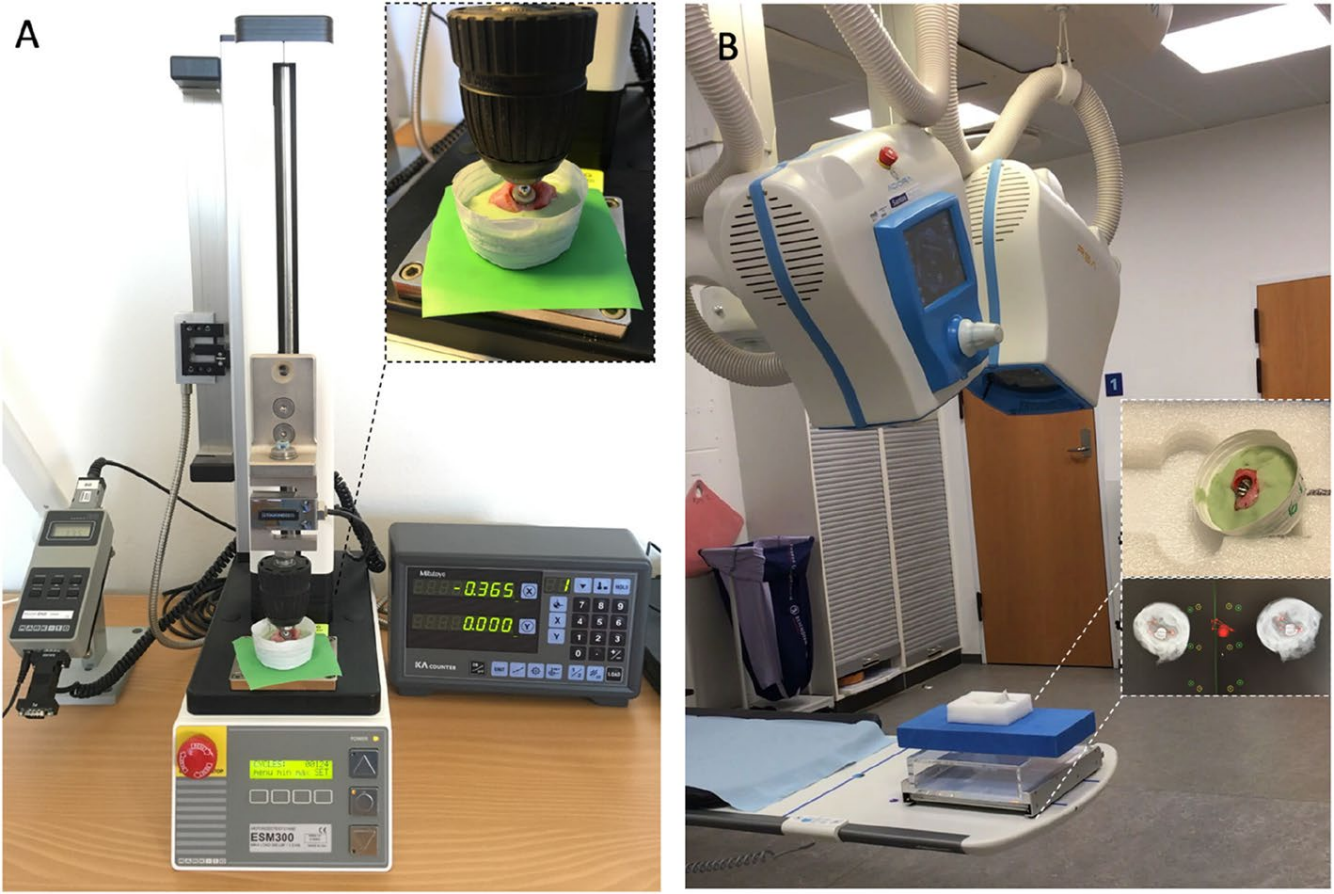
A The motorized Mark-10 test stand mounted with a 5 mm head-neck segment to apply a compression load on the cup during testing. B The DR RSA set-up with two ceiling-mounted x-ray tubes used for recording stereo-radiographs of the cup and bone-markers
